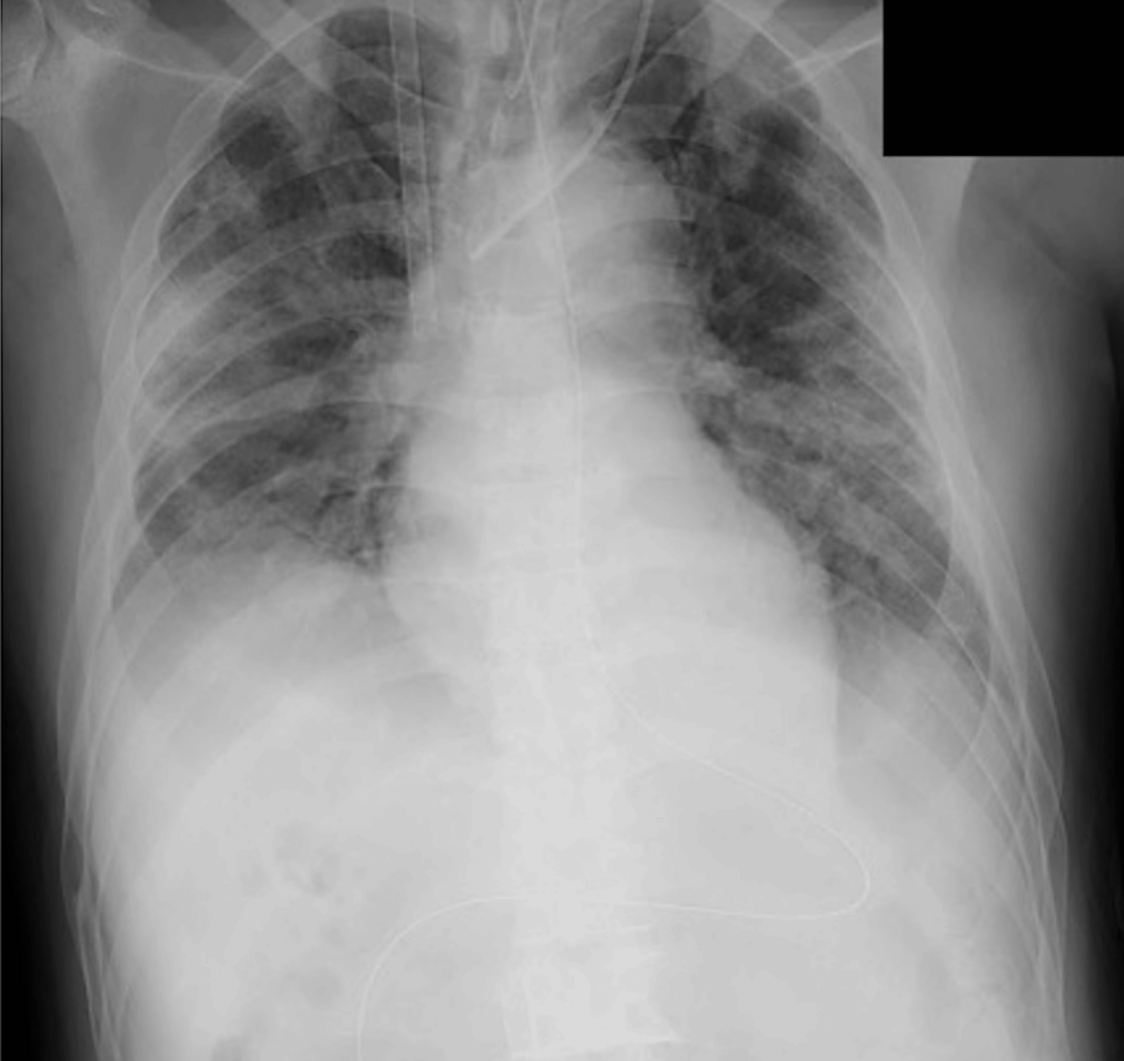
Chest radiography after cannulation
